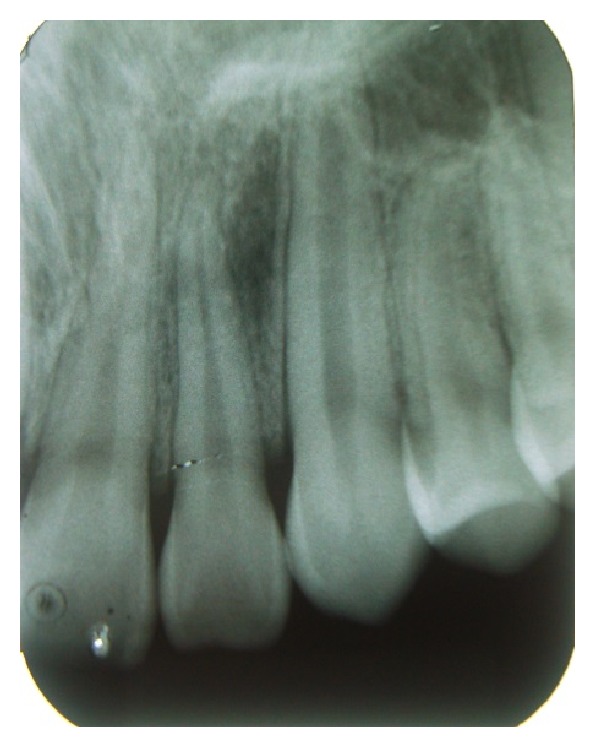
Radiographic control after four years without signs of abnormality.
